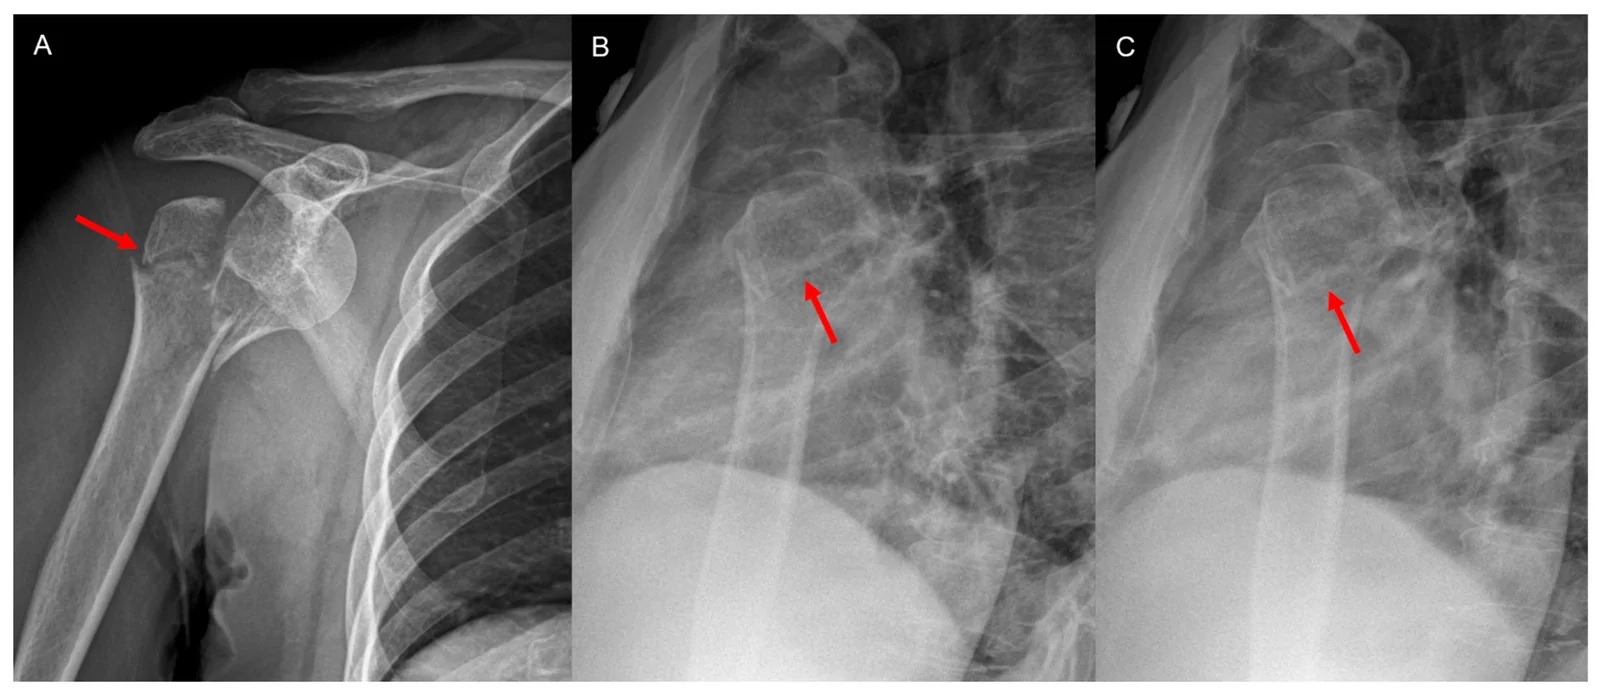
Shoulder radiographs of a 35-year-old male patient with preoperative humeral head fracture-dislocation. (A) Anteroposterior shoulder radiograph showed the humeral head fracture-dislocation (red arrow). (B) Transthoracic lateral radiograph obtained using the routine free-breathing method, showing relatively poor conspicuity of the fracture because of the thoracic soft-tissue superimposition and respiratory motion blurring, which could easily be mistaken for the margin of a rib. (C) Corresponding lateral radiograph acquired using the deep-inspiration breath-hold method, demonstrating improved image contrast and sharper delineation of the fracture and adjacent cortical margins (red arrow) when compared with those in (B).
